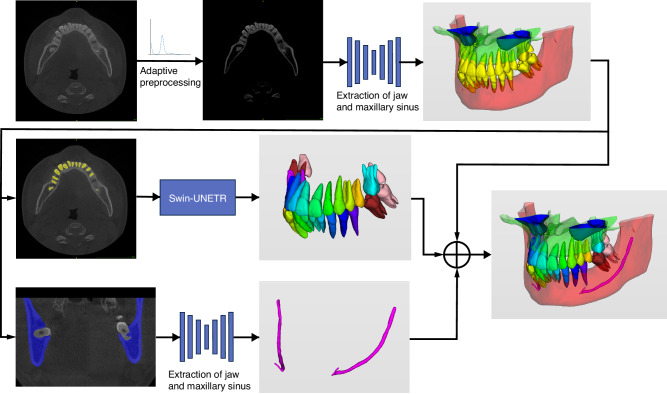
Overview of our proposed artificial intelligence system for segmenting individual teeth, maxillary and mandibular bones, maxillary sinus, and mandibular neural tube from CBCT images (The input to the system is a 3D CBCT scan of the patient; a uniform image is first obtained using a CBCT image adaptive preprocessing algorithm, then processed by a bone extraction, neural tube extraction and tooth instance segmentation network, and finally a mask containing all structures to be segmented is output)
